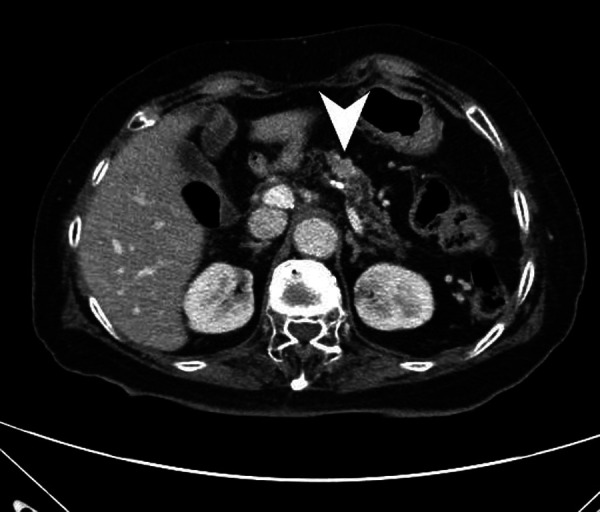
Abdominal CT revealed a big lesion (arrowhead) at the remnant pancreas body.
